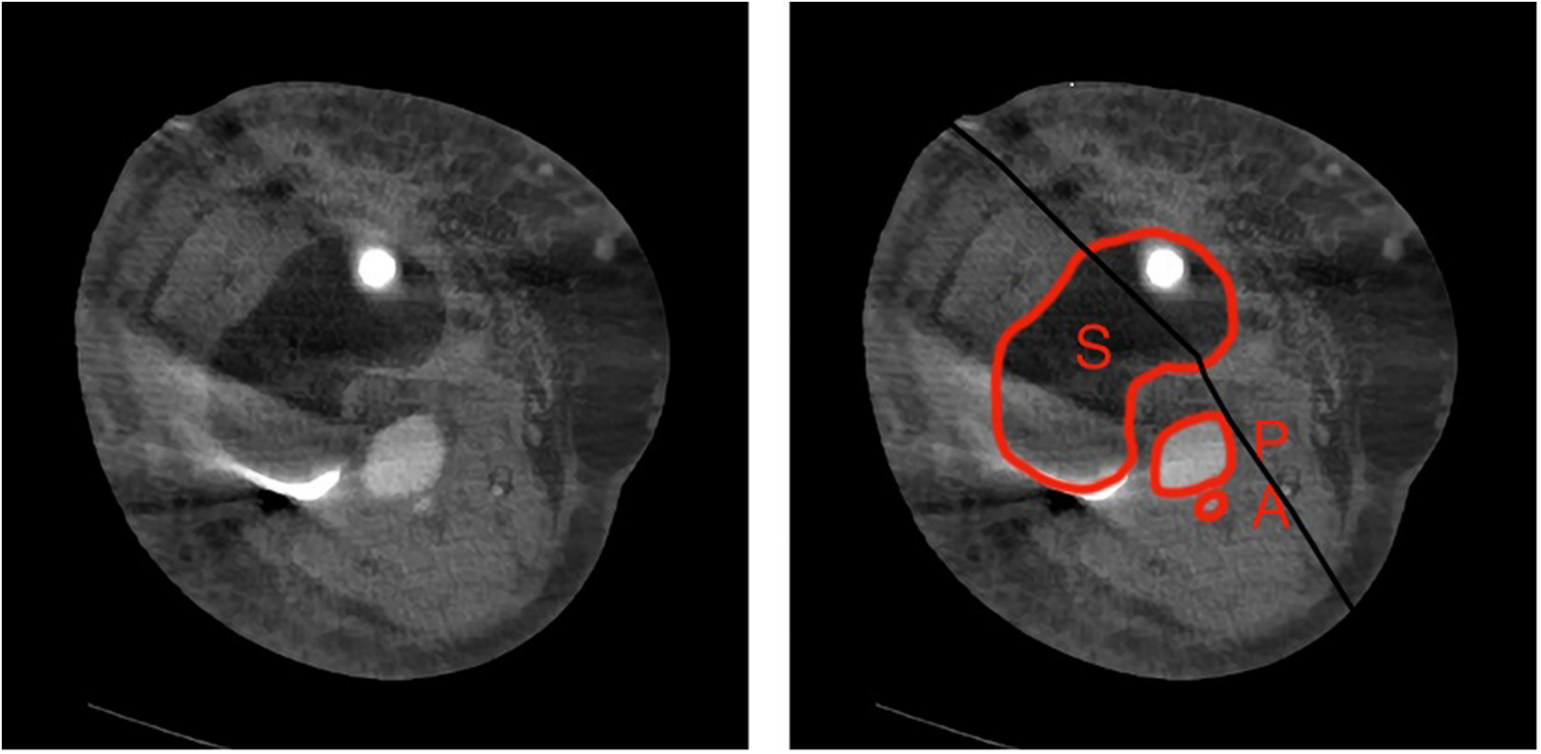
Preoperative axial CT angiography image at the level of the joint space showing the pseudoaneurysm directly ventral of the popliteal artery. A, popliteal artery; P, pseudoaneurysm; S, spacer of the knee prosthesis.
